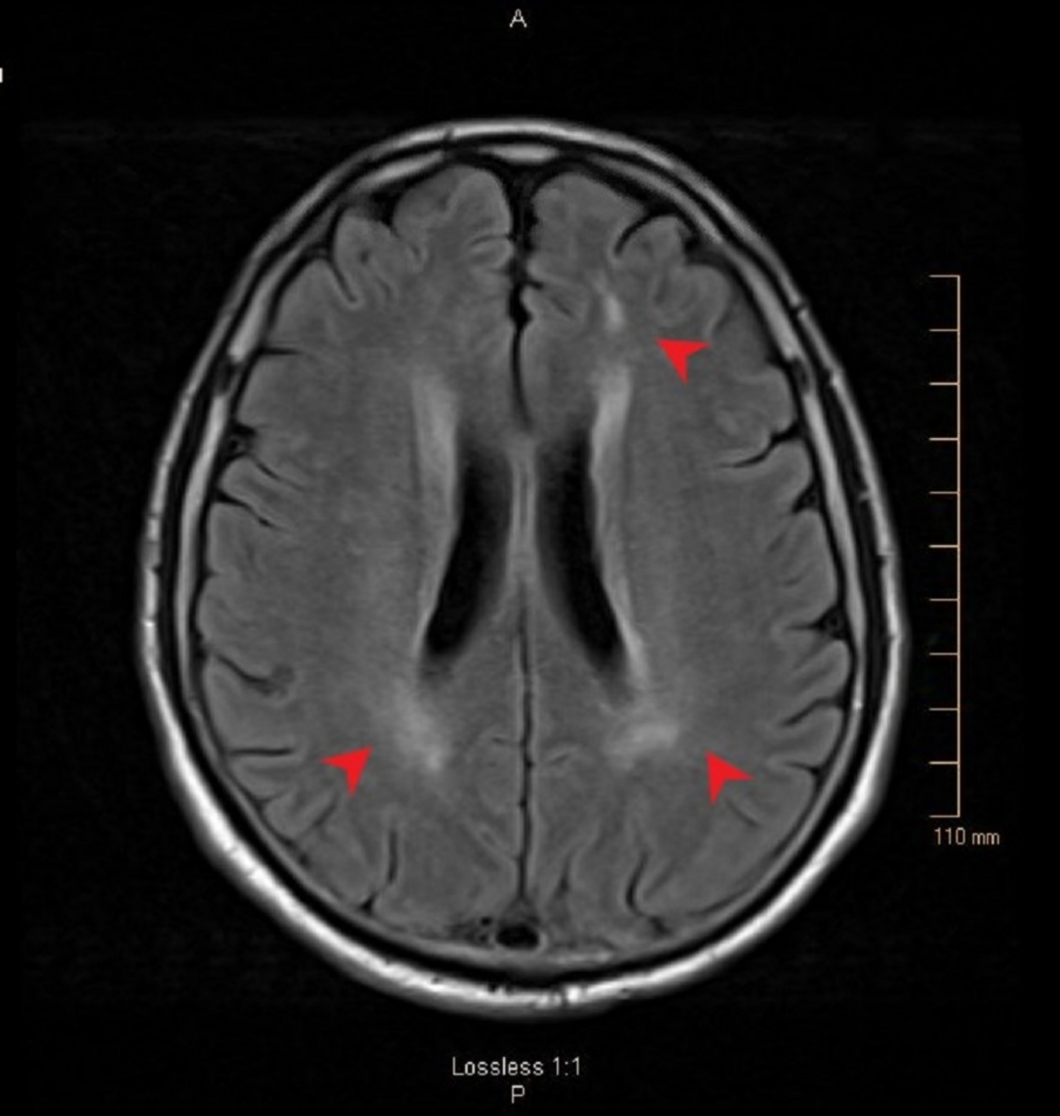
MRI on admission shows periventricular white matter changes on FLAIR sequence (red arrowhead). MRI: Magnetic resonance imaging; FLAIR: Fluid-attenuated inversion recovery.
